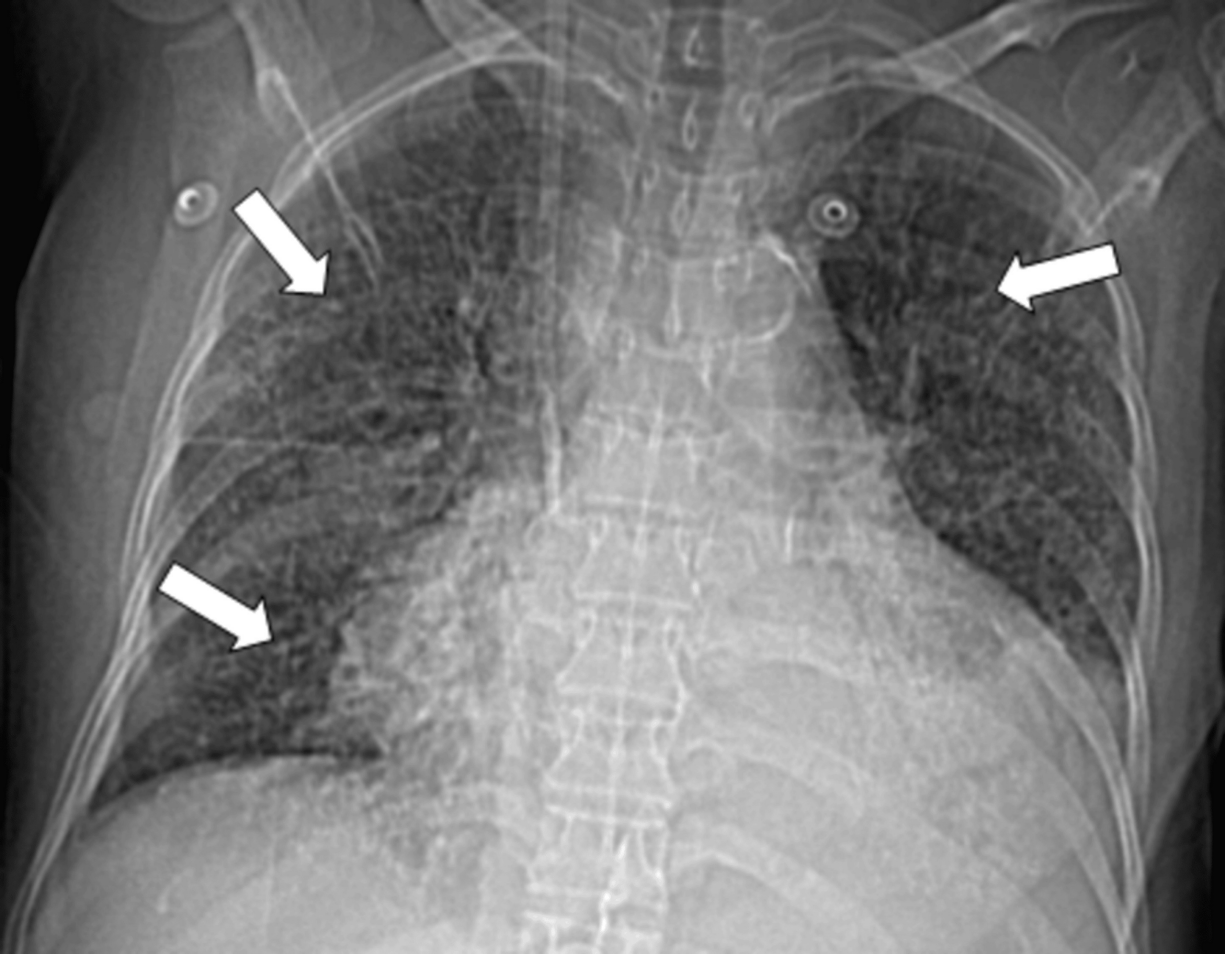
Chest X-ray AP view shows multiple tiny hyperdense foci in bilateral lung fields (indicated by arrows). AP: Anteroposterior
